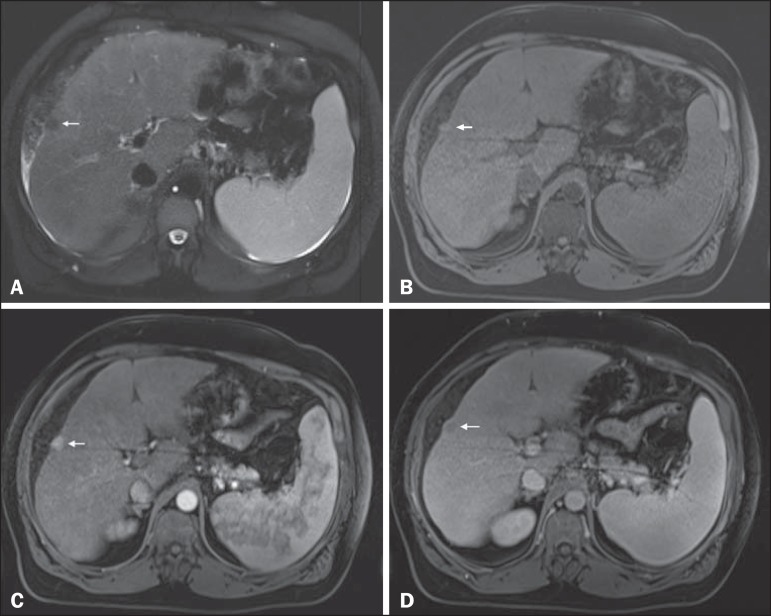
Small* HCC. Axial SSFSE T2-weighted image, with fat
